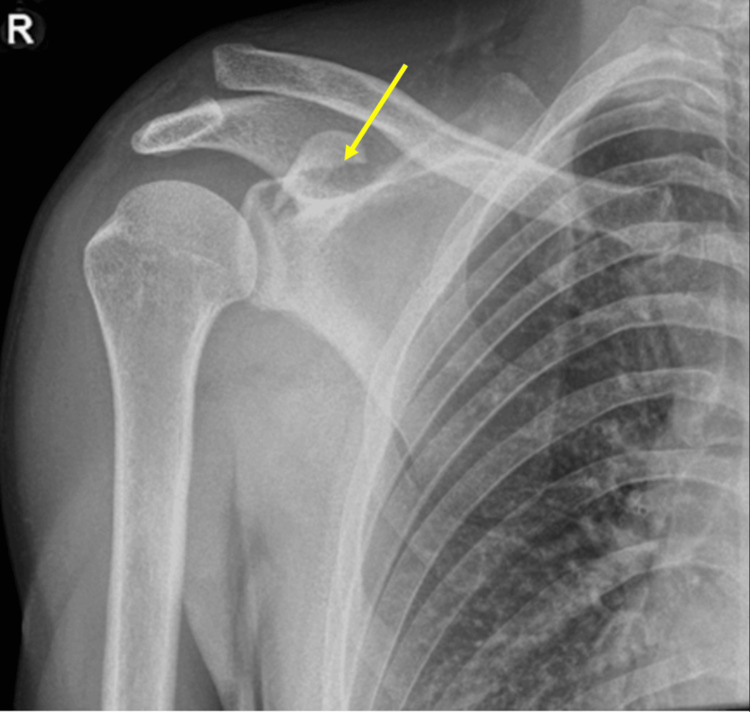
AP shoulder radiograph showing AC joint displacement with an associated coracoid fracture fragment The fractured coracoid process is indicated by the yellow arrow in this image. AC: acromioclavicular; AP: anterior-posterior
